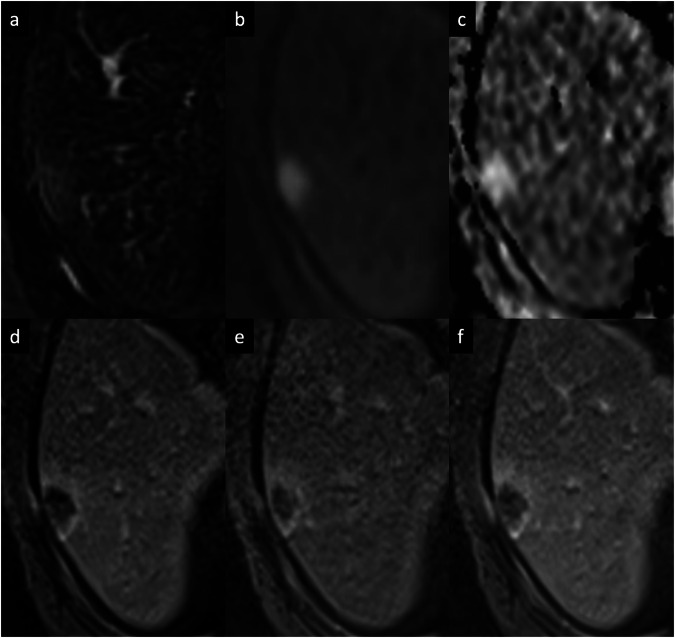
Solitary necrotic nodule in a 52-year-old man. Extracellular contrast agent-enhanced MRI shows a subcapsular lesion in the right hepatic lobe, (a) slightly hyperintense on T2-weighted images, (b) high SI on DWI, and (c) with high values on ADC map. d The lesion demonstrates a rim APHE that persisted on portal venous (e) and delayed (f) phases. A biopsy of the lesion confirmed the diagnosis of a solitary necrotic nodule in the context of homogenous and moderate hepatic steatosis
